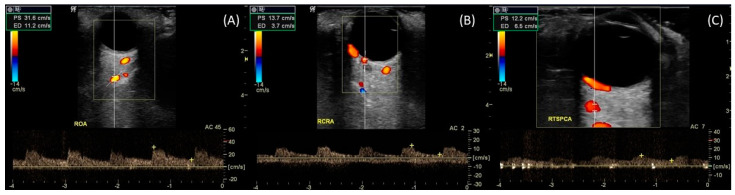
Using the B-scan mode to identify ocular structures. Color Doppler is applied to record the Doppler waveforms: (A) Ophthalmic Artery, (B) Central Retinal Artery, (C) Temporal Short Posterior Ciliary Arteries.
